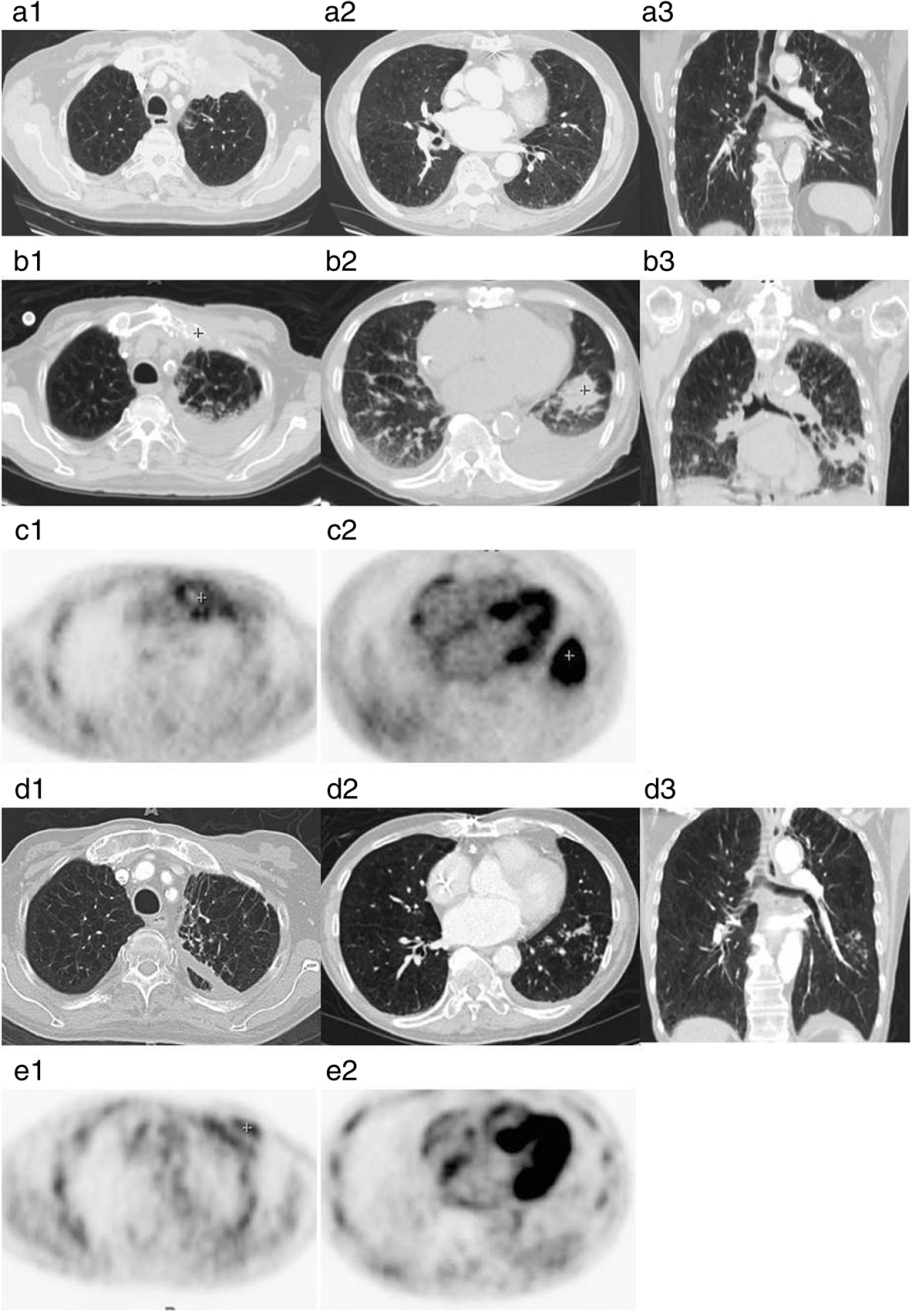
a1-a3: Baseline CT images of the left upper chest wall tumor, a large 6 cm × 9 cm LUL mass invading through the left anterior chest wall into ribs as (four months prior to pleural effusion); b1-b3: Response in the left upper lobe chest wall mass, but appearance of a new LLL nodular mass as well as pleural effusion (March); c1-c2:^18^FDG-PET image demonstrated intense FDG activity fusing to 3.3 × 4 cm left lower lobe mass with SUV max 9.9, but minimal left upper lobe chest wall uptake (March); d1-d3:Chest CT scan post-fluconazole therapy (five months post-fluconazole therapy) showed that Left lower lobe mass has significantly diminished in size. Right pleural effusion had resolved; e1-e2: 18FDG-PET image follow-up post PC therapy shows resolution of LLL uptake, with some minimal chest-wall uptake (eight months post-fluconazole therapy)
